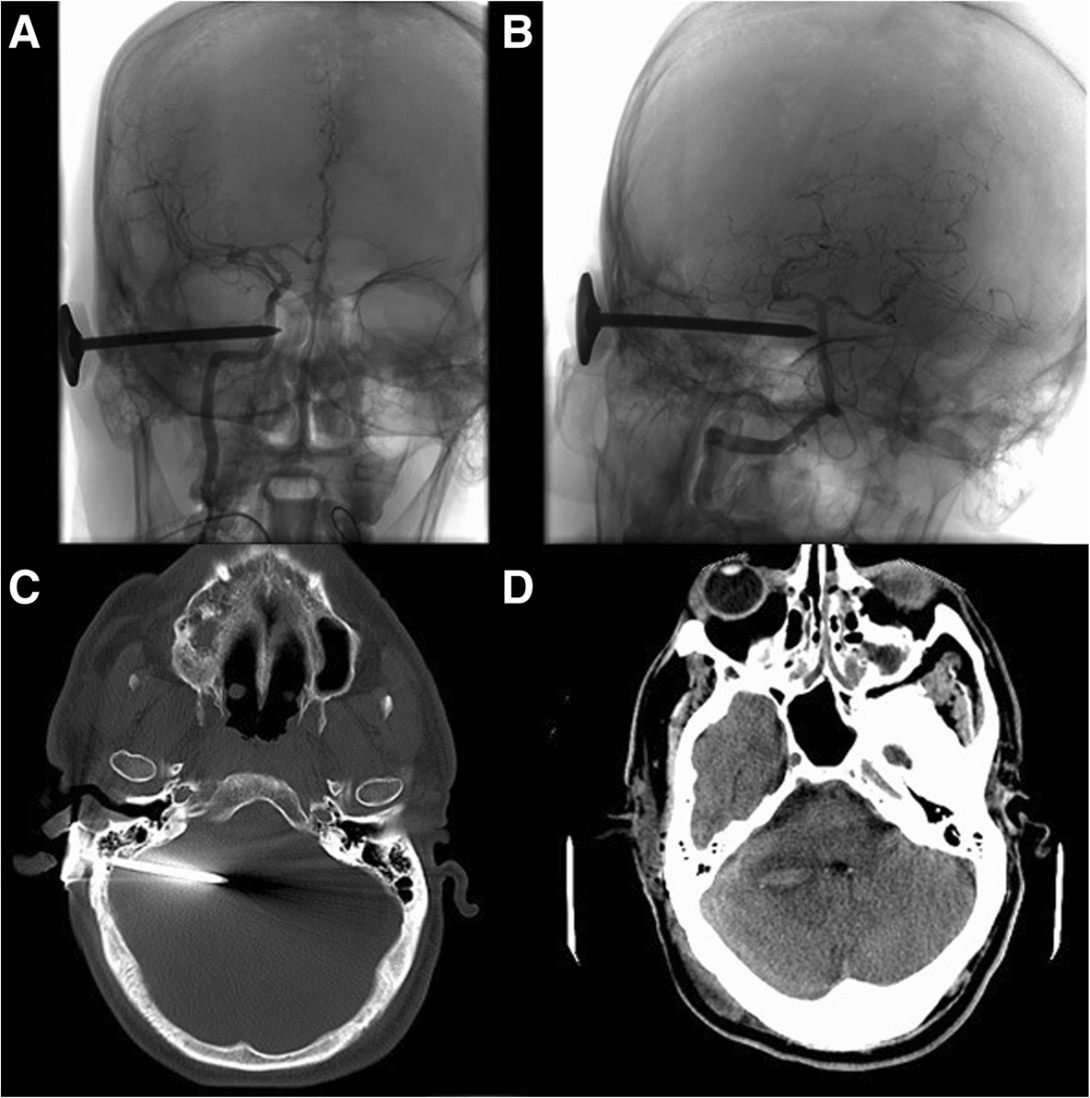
Cerebral angiogram. a Right internal cerebral artery. b Right vertebral artery. The metallic foreign body transects the superior aspect of the right transverse sinus as it merges into the right sigmoid sinus. There is no active extravasation of contrast or evidence of major arterial compromise. c Preoperative computed tomography of the patient’s head (bone window). d Postoperative computed tomography of his head. Small amount of hemorrhage noted along the track of the removed foreign body from the right posterior fossa and evidence of a small intraventricular hemorrhage
